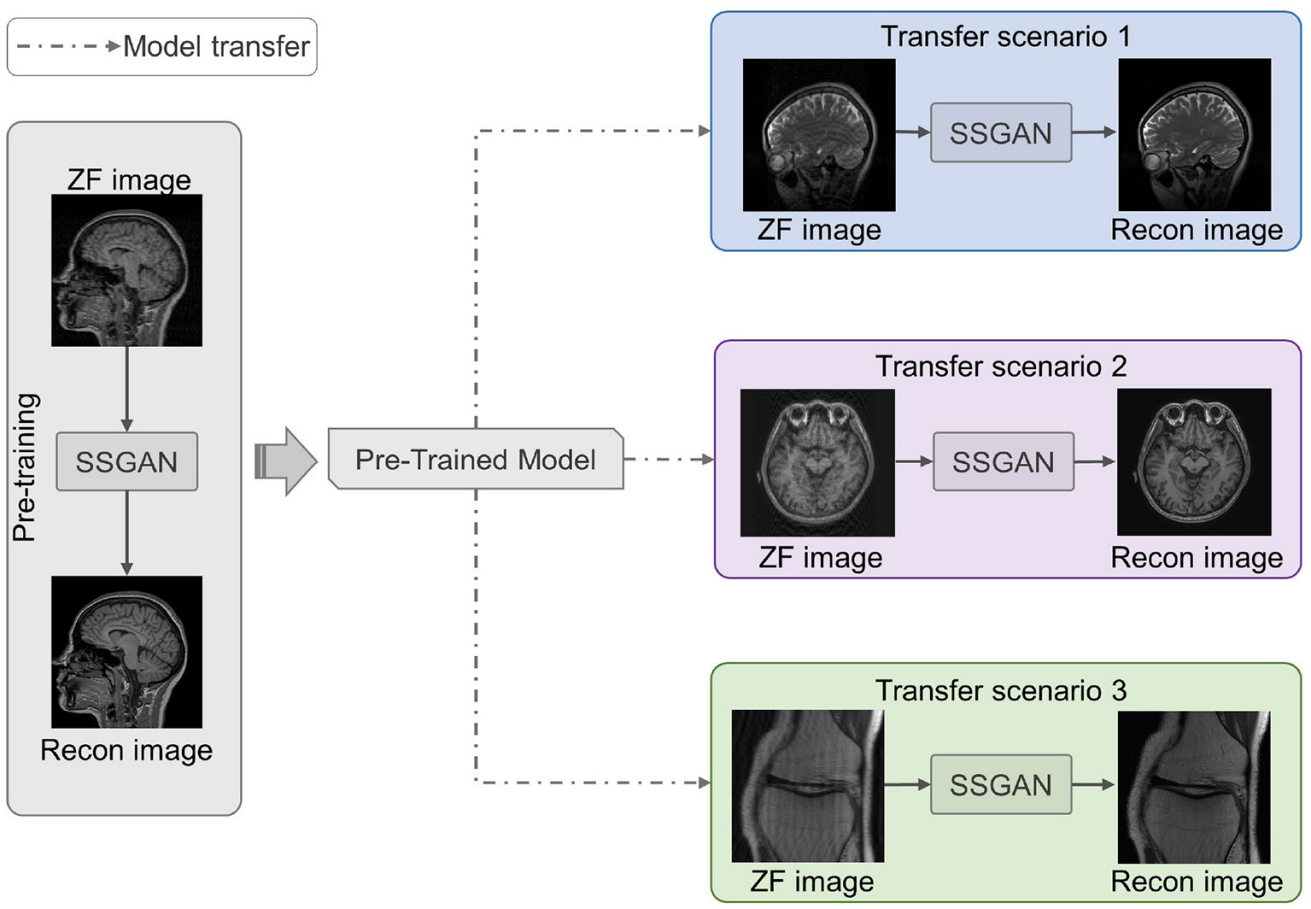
Three transfer scenarios of the pre-trained SSGAN. The gray part shows SSGAN was pre-trained on the IXI dataset, then transferred to reconstruct images with different contrasts (blue part, scenario 1: T1-weighted to T2-weighted), slicing directions (purple part, scenario 2: axial planes to sagittal planes), and anatomical structures (green part, scenario 3: brain to knee).
